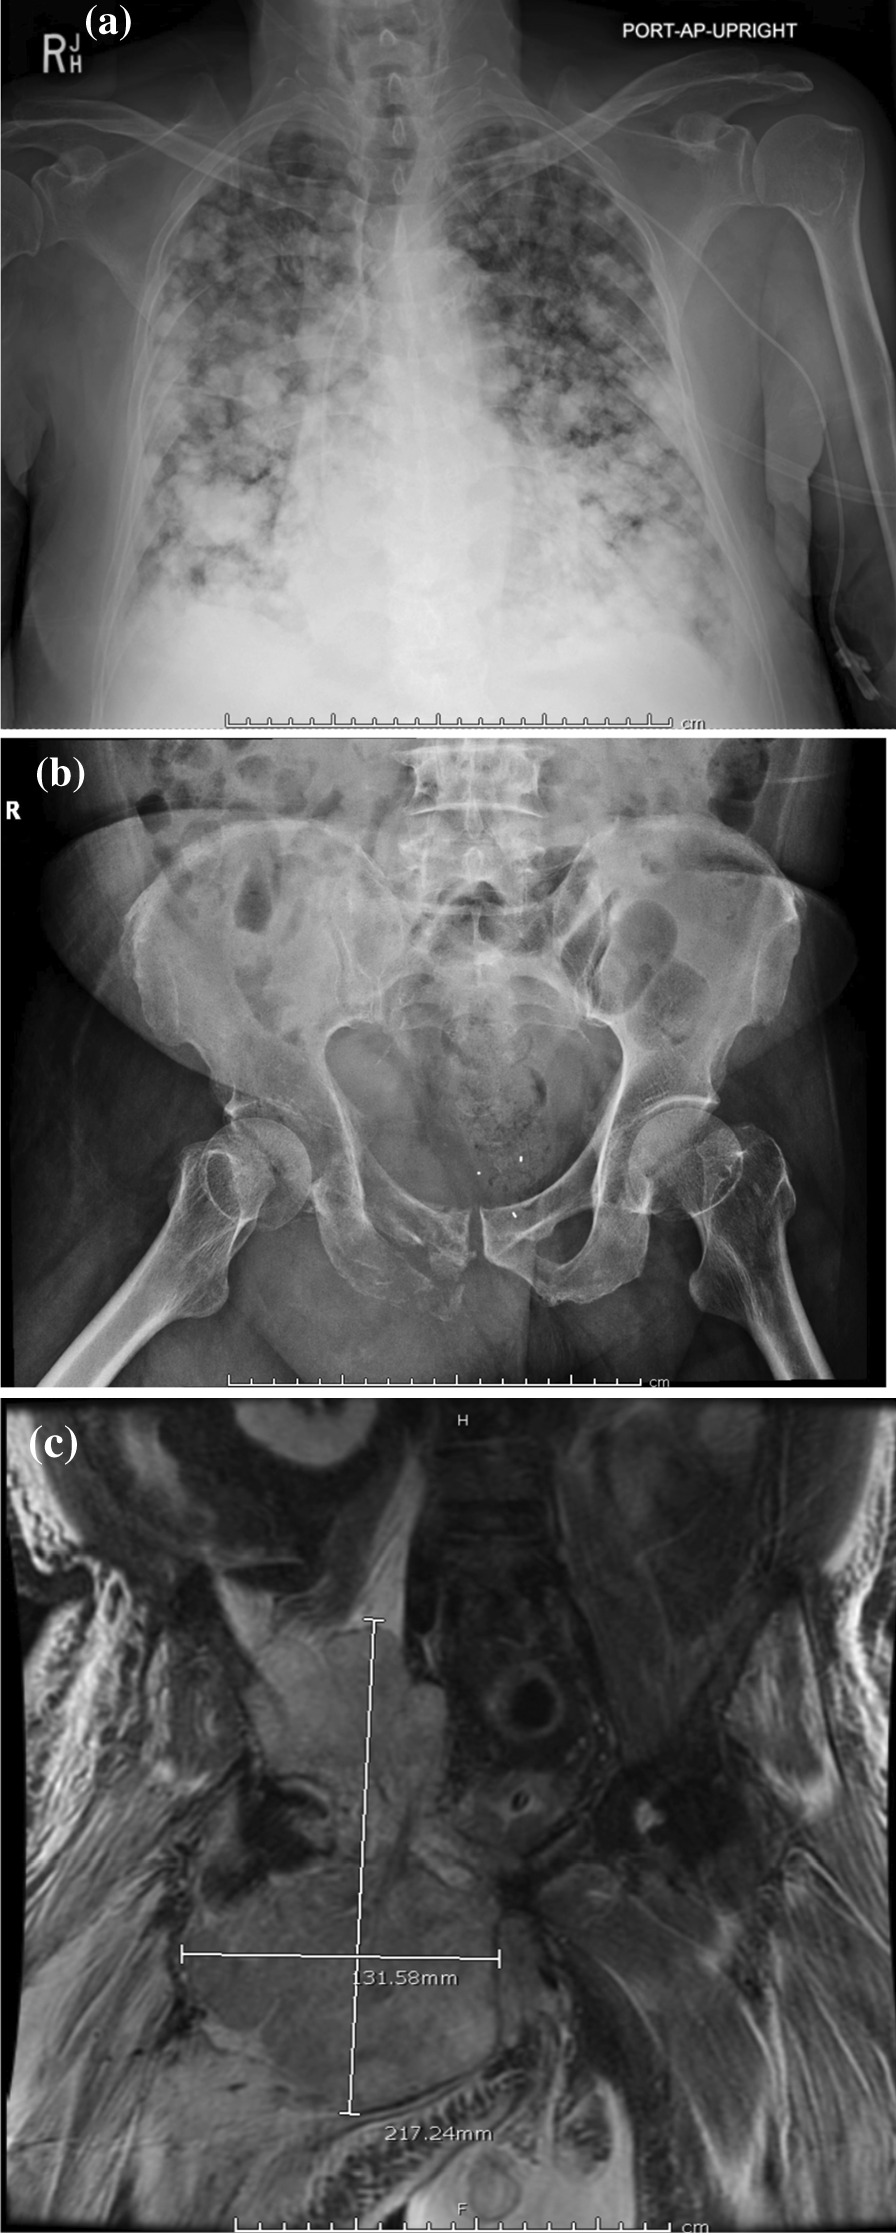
a Anterioposterior (AP) chest X-ray demonstrating innumerable pulmonary nodules and masses consistent with metastatic disease. b AP pelvis X-ray demonstrating pathological fractures involving the right superior and inferior pubic rami, right acetabulum, and pubic symphysis. c Representative coronal MRI cross section demonstrating a large multilobulated, irregular mass involving the right hemipelvis with intraosseous and soft tissue components. The mass demonstrates predominantly low T1 signal and heterogeneous STIR signal measuring (in unshown cross-sections) 15.3 × 21.7 × 12.7 cm. The mass completely replaces the marrow space of the right acetabulum extending into the ilium, pubis involving the pubic symphysis, and ischium with associated pathologic fractures and destruction of the cortex. There is mass effect upon the right iliopsoas musculature with likely invasion. The same process is seen within the left hip rotators and adductors.
